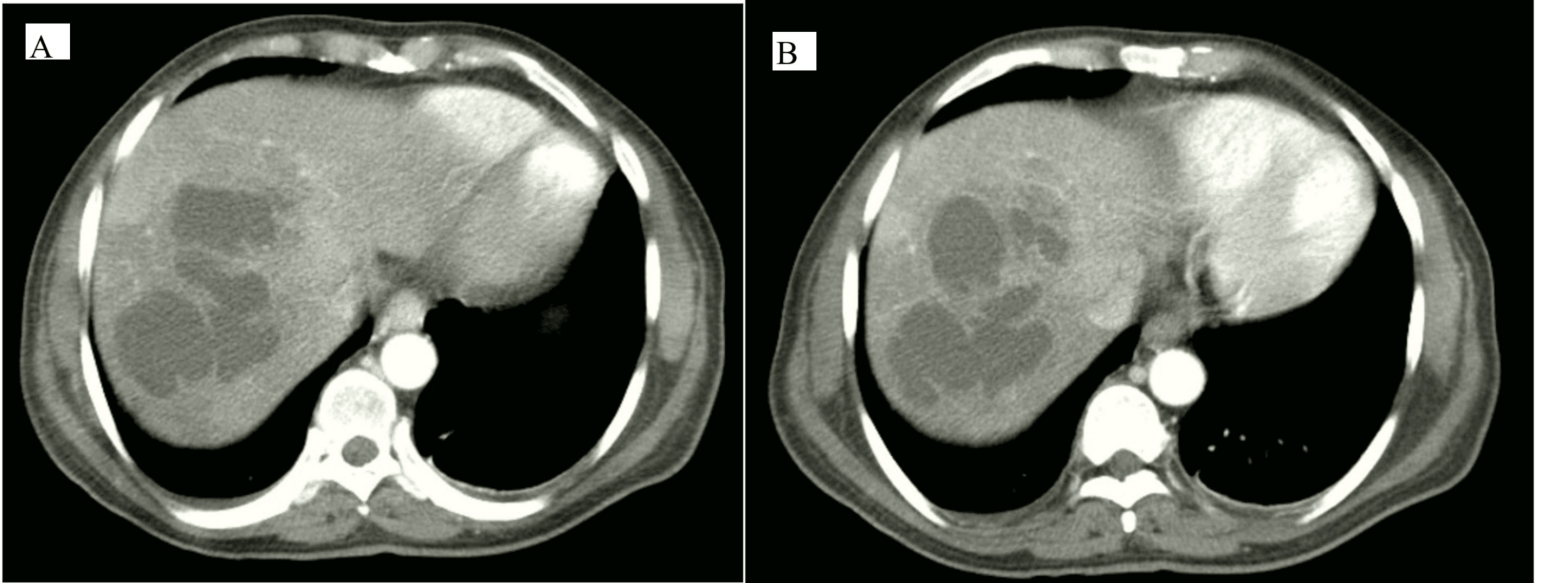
CT scan of the abdomen showed a liver lesion. Both images of axial CT with different levels show multiple loculated coalescent hepatic cystic lesions implicating the right hepatic lobe (segment VIII, VII) with a 13 cm CC diameter.
